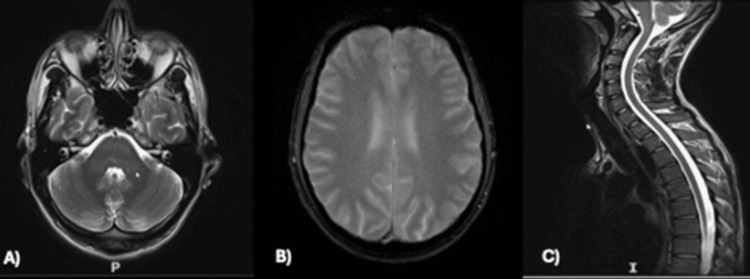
Normal magnetic resonance imaging of the patient's brain and spine, with no evidence of demyelination, hemorrhage, or infarct. (A) MRI head T2-weighted sequence, (B) MRI head susceptibility-weighted imaging (SWI) sequence, and (C) MRI spine.
